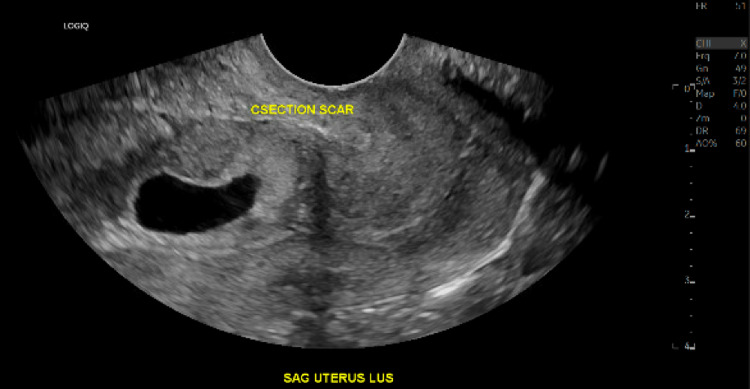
Transvaginal grayscale ultrasound image demonstrating an intrauterine gestational sac in the lower uterine segment at the level of the cesarean section scar.
